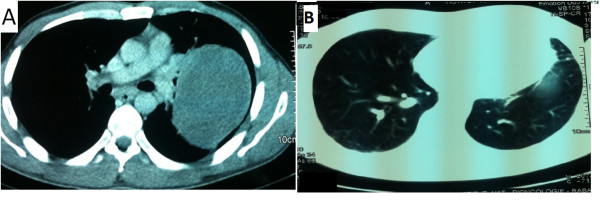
Chest computed tomography scan. (A): lesional process of the left upper lobe before treatment. (B): No signs of recurrence after 2 years.
